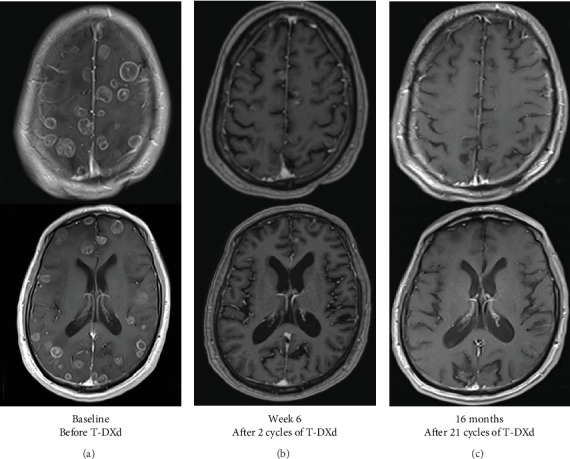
Axial view of MRI brain, postcontrast, showing innumerable metastases at (a) time of presentation after 1 cycle of pemrolizumab and prior to T-DXd, (b) decrease in size and number of lesions after two cycles of T-DXd, and (c) ongoing response after 16 months (21 cycles) of T-DXd. MRI, magnetic resonance imaging; T-DXd, trastuzumab deruxtecan.
